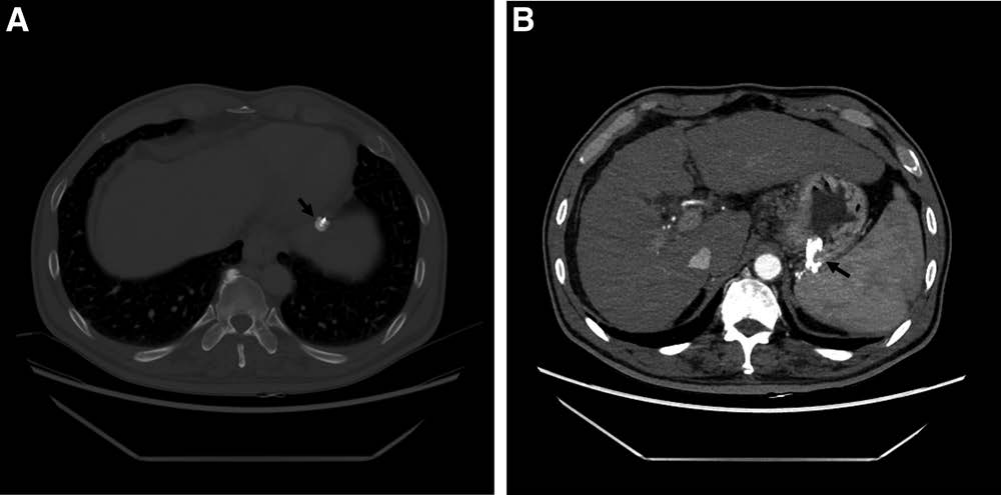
(A) Vascular plug (arrow) is placed in the embolized transverse portion of left inferior phrenic vein. (B) Follow-up CT image showing complete disappearance of the gastric varix. Histoacryl (arrow) injected endoscopically can also be observed. CT = computed tomography.
